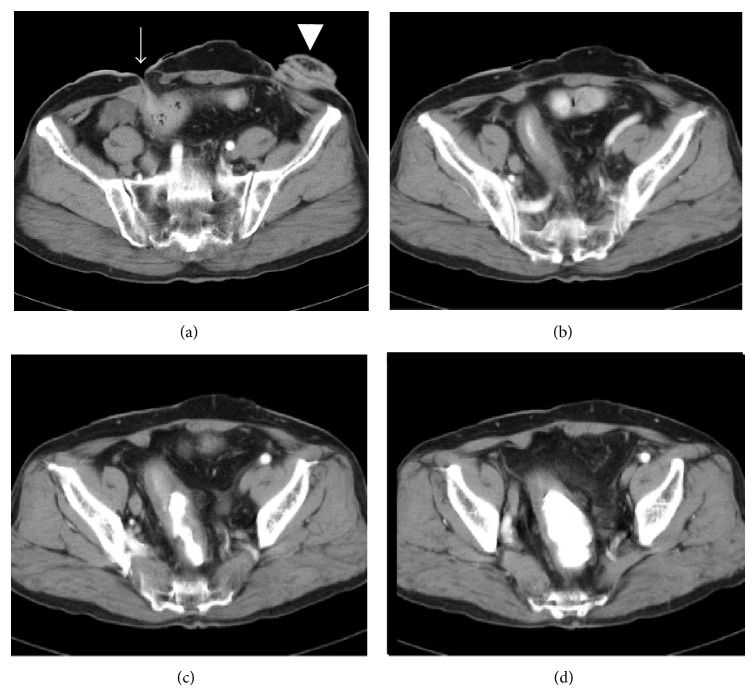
CT performed after surgery indicated that the rectal dilation had resolved ((a), (b), (c), and (d)). A mucous fistula of the upper rectum was created in the right lower abdomen (arrow). A sigmoid divided colostomy was created in the left lower abdomen (arrow head).
